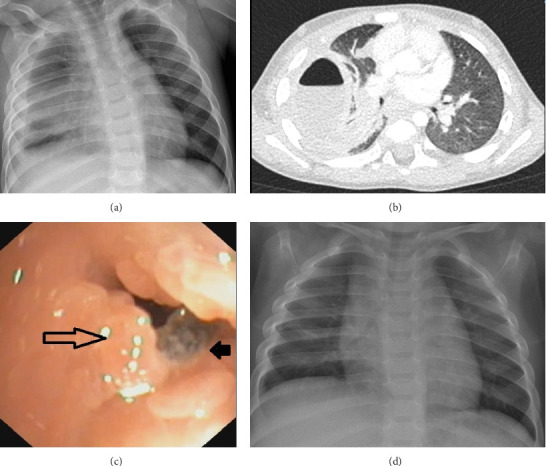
(a) The chest plain radiogram on admission for the first case showing a right lower and middle lobe infiltrate with a pleural effusion. (b) The CT scan for the first case after readmission demonstrating a large, thick-walled effusion on the right with an air-fluid level and an adjacent infiltrate in the right lower lobe. (c) The image from the bronchoscopy in the first case. The black arrow points to the foreign body, and the open arrow shows the granuloma. (d) The chest plain radiogram for the first case after removal of the foreign body and subsequent recovery.
